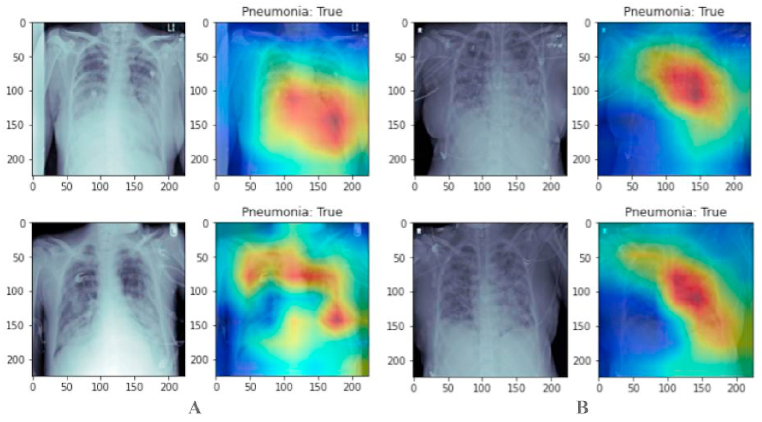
Classification result of chest x-rays for positive pneumonia using our capsule networks cluster model. In Panel “A” the right side of images are original while the left sides of images are processed through our model. In Panel “B” the right side of images are original from data side while the left side shows detected penuomenia in them.
